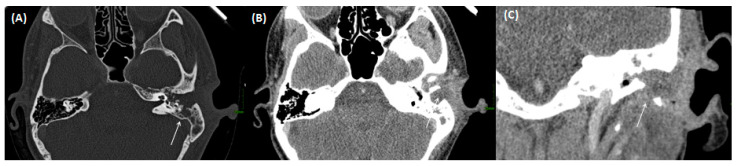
CT of a 26-year-old female patient with axial images using bone (A) and soft tissue (B) windowing, and coronal orientation using soft tissue windowing (C). A cholesteatoma is demarked as a soft tissue focus within the EAC with associated erosions at the border to the mastoidal cells.
